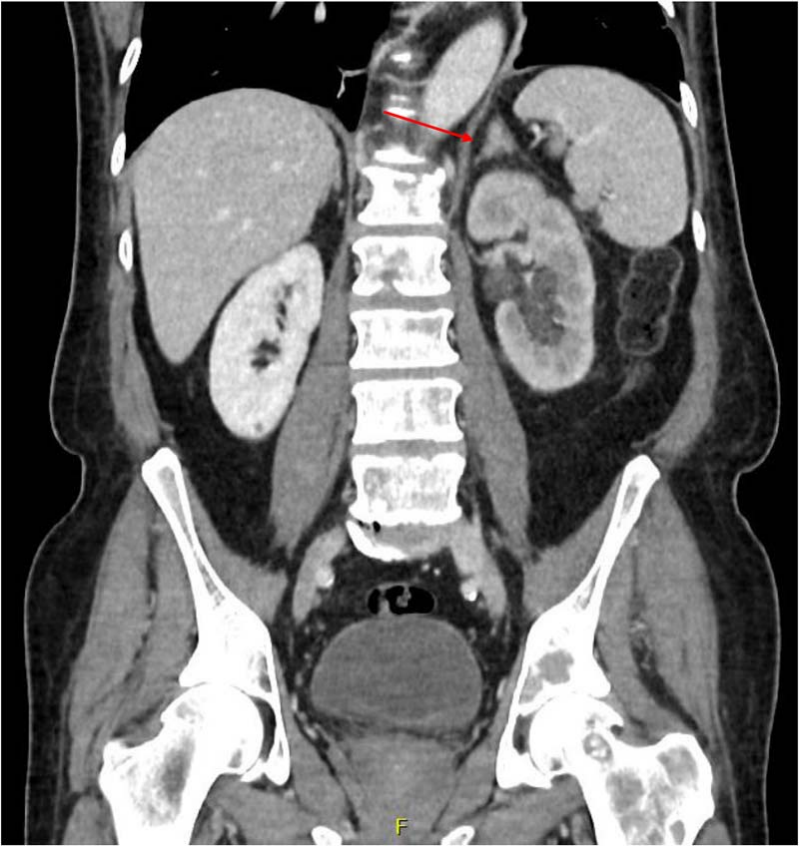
Coronal CT abdomen and pelvis scan with contrast demonstrating left-sided hydronephrosis and left-sided adrenal mass (red arrow).
